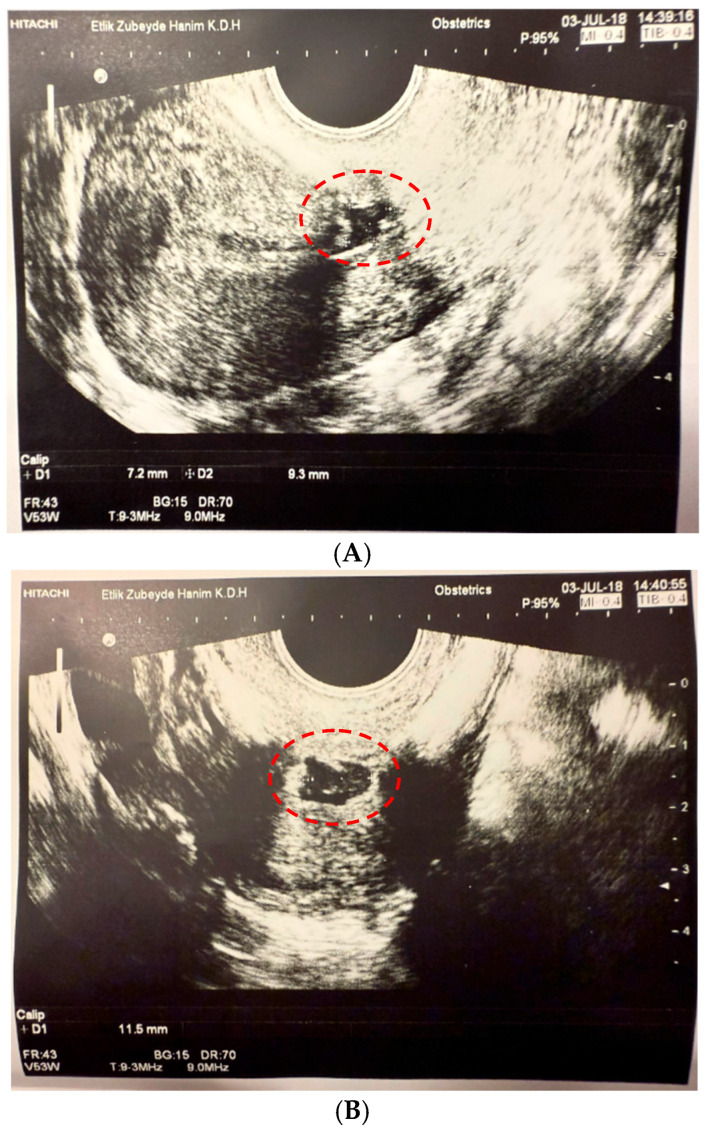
Calculation of Isthmocele Volume in Ultrasonography ((A) Height-Depth Measurement (D1*D2), (B) Width Measurement (D1)). Height-Depth Measurement is indicated as D1*D2 in panel (A), and Width Measurement is indicated as D1 in panel (B) (as described in the figure legend). The measured areas are highlighted with red circles on the images.
